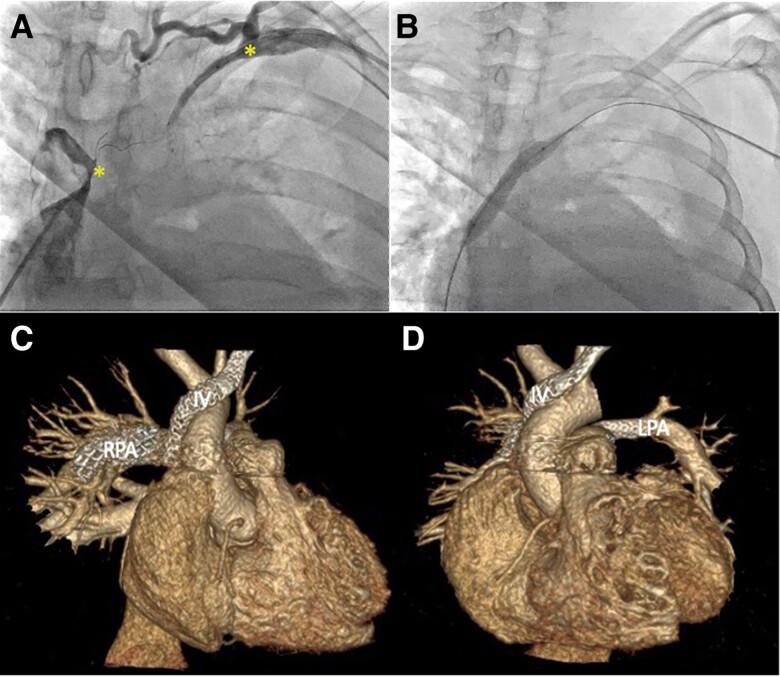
Venous angiography. (*A*) shows total obstruction at the level of the superior vena cava and the innominate vein (asterisks). (*B*) Balloon dilation at the level of the innominate vein. Three-dimensional reconstruction of cardiac computed tomography. (*C*) Image shows stent in the right pulmonary artery (RPA) and innominate vein (IV). (*D*) A stent is evident in the left pulmonary artery (LPA) and IV.
